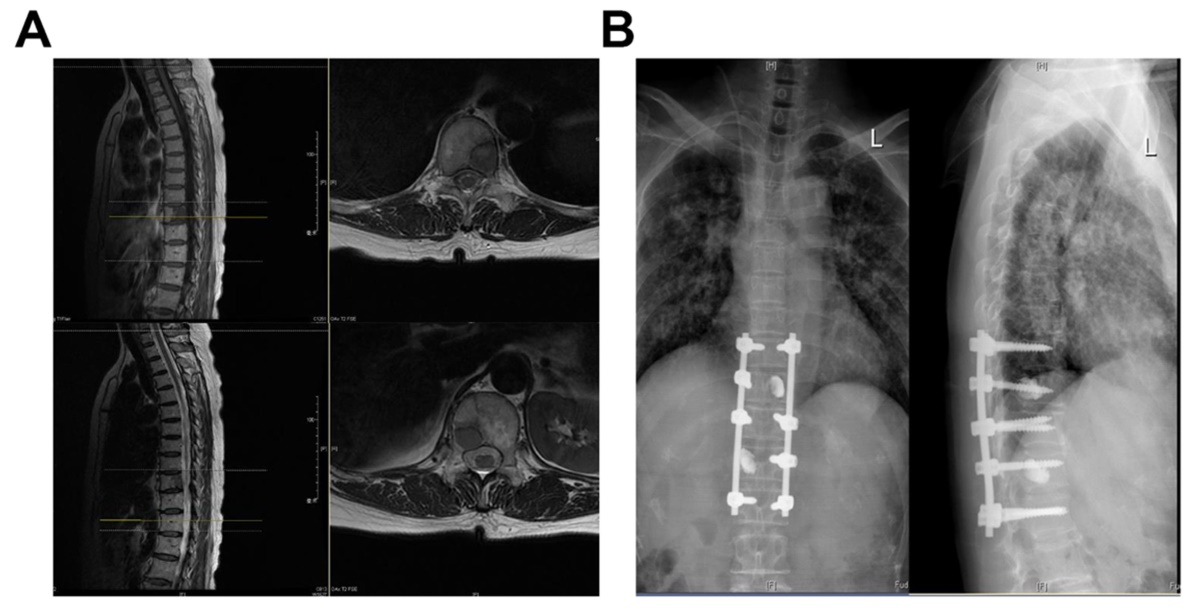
Case: Female, 60 years old, thoracic vertebrae 10 and 12 with lung cancer metastases. (A) Preoperative MRI; (B) Three Months Postoperative X-Ray.
